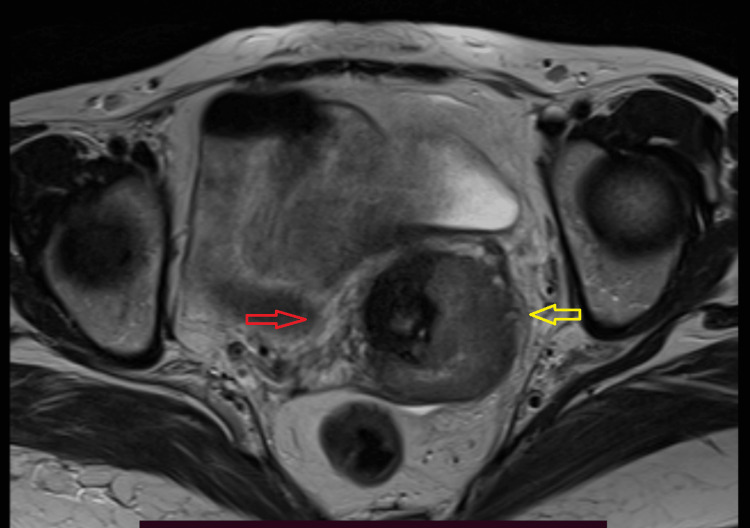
The MRI image showing involved and uninvolved parametrium in an early cervical cancer patient The yellow arrow shows an involved parametrium with loss of the hypointense ring. The red arrow shows an uninvolved parametrium with an intact hypointense ring.
